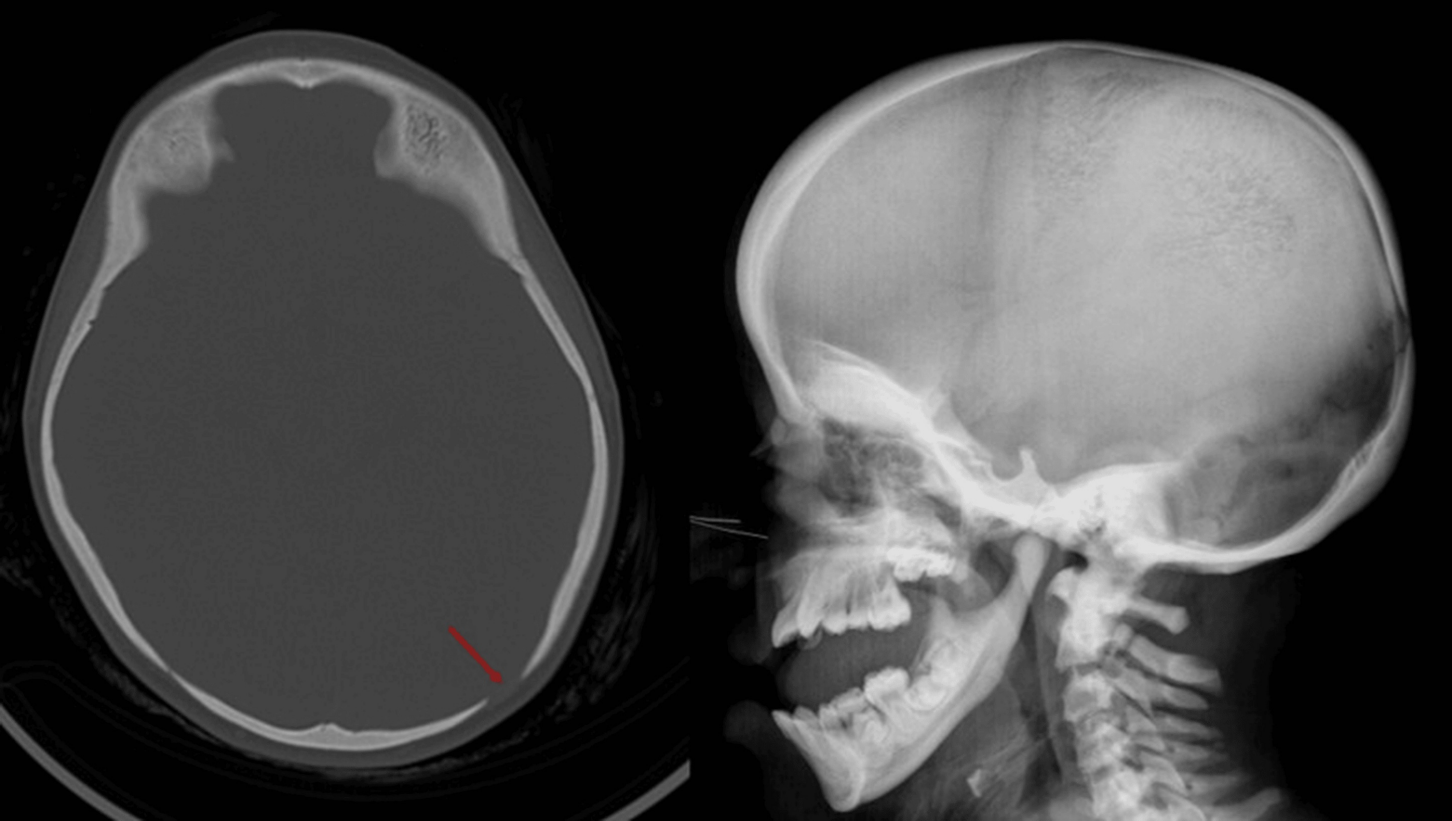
Mid-axial CT section demonstrating a widened gap between bone structures near the lambdoid lesion, with corresponding sagittal X-ray view. Arrow highlights the suture irregularity.
